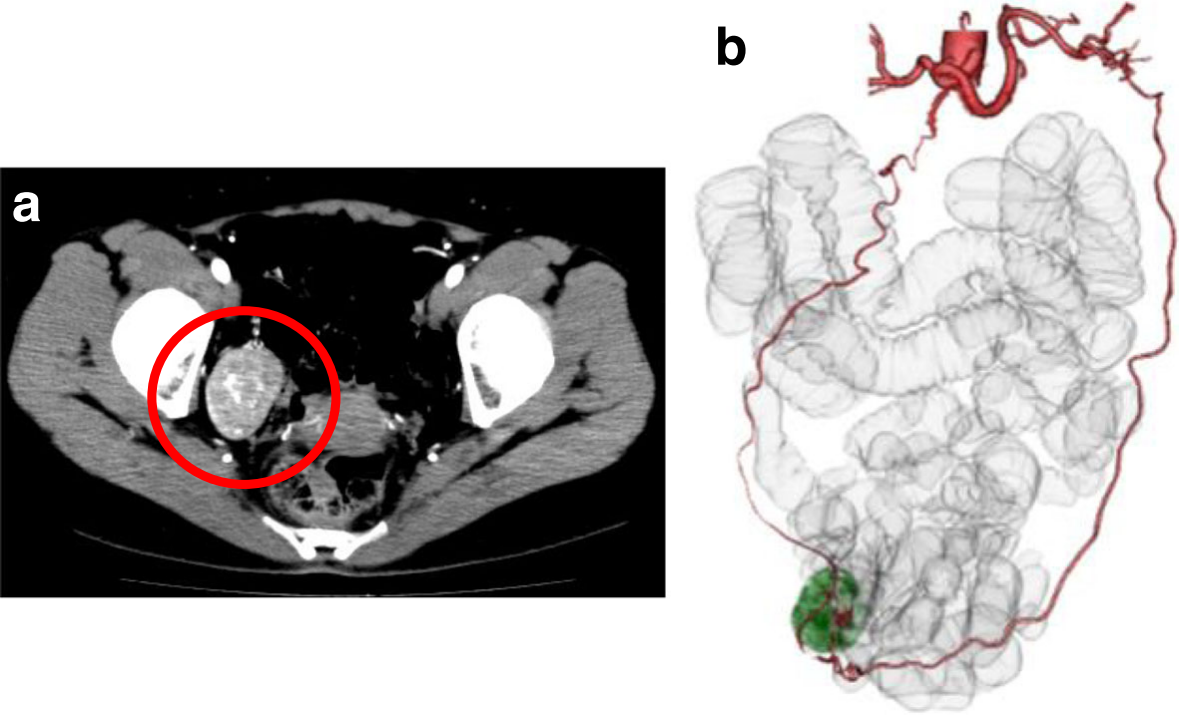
Contrast-enhanced CT image findings. a A 45-mm-large tumor mass on the right side of the pelvic floor. Inhomogeneous mineralization which was somewhat borderless was recognized inside. b Clear nutrient vessels originating from the splenic artery were considered omentum branches
